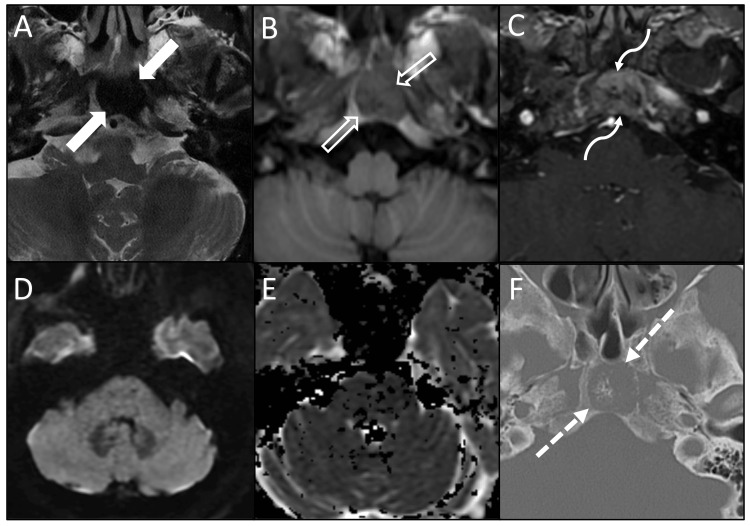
Multimodality imaging features of a clival mass: MRI and CT characteristics Additional axial MRI images of the clival mass demonstrate complete loss of signal on T2 (A, solid arrows), T1 marrow replacement (B, open arrows), heterogeneous enhancement (C, curved arrows), and no signal on DWI or ADC (D, E). Comparison axial CT demonstrates the clival lesion to be lytic with residual internal bony trabecula (F, dashed arrows).
